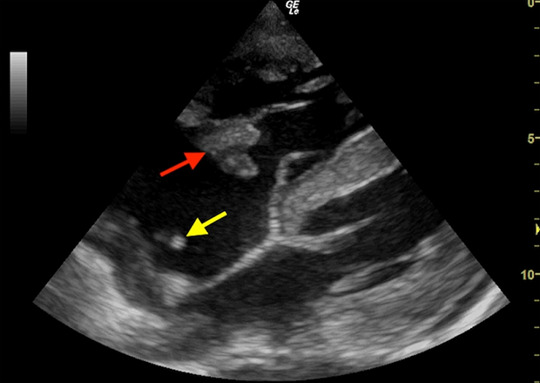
point-of-care ultrasound image demonstrating a tricuspid valve vegetation (red arrow) and the tip of a central venous catheter in the right atrium (yellow arrow)
